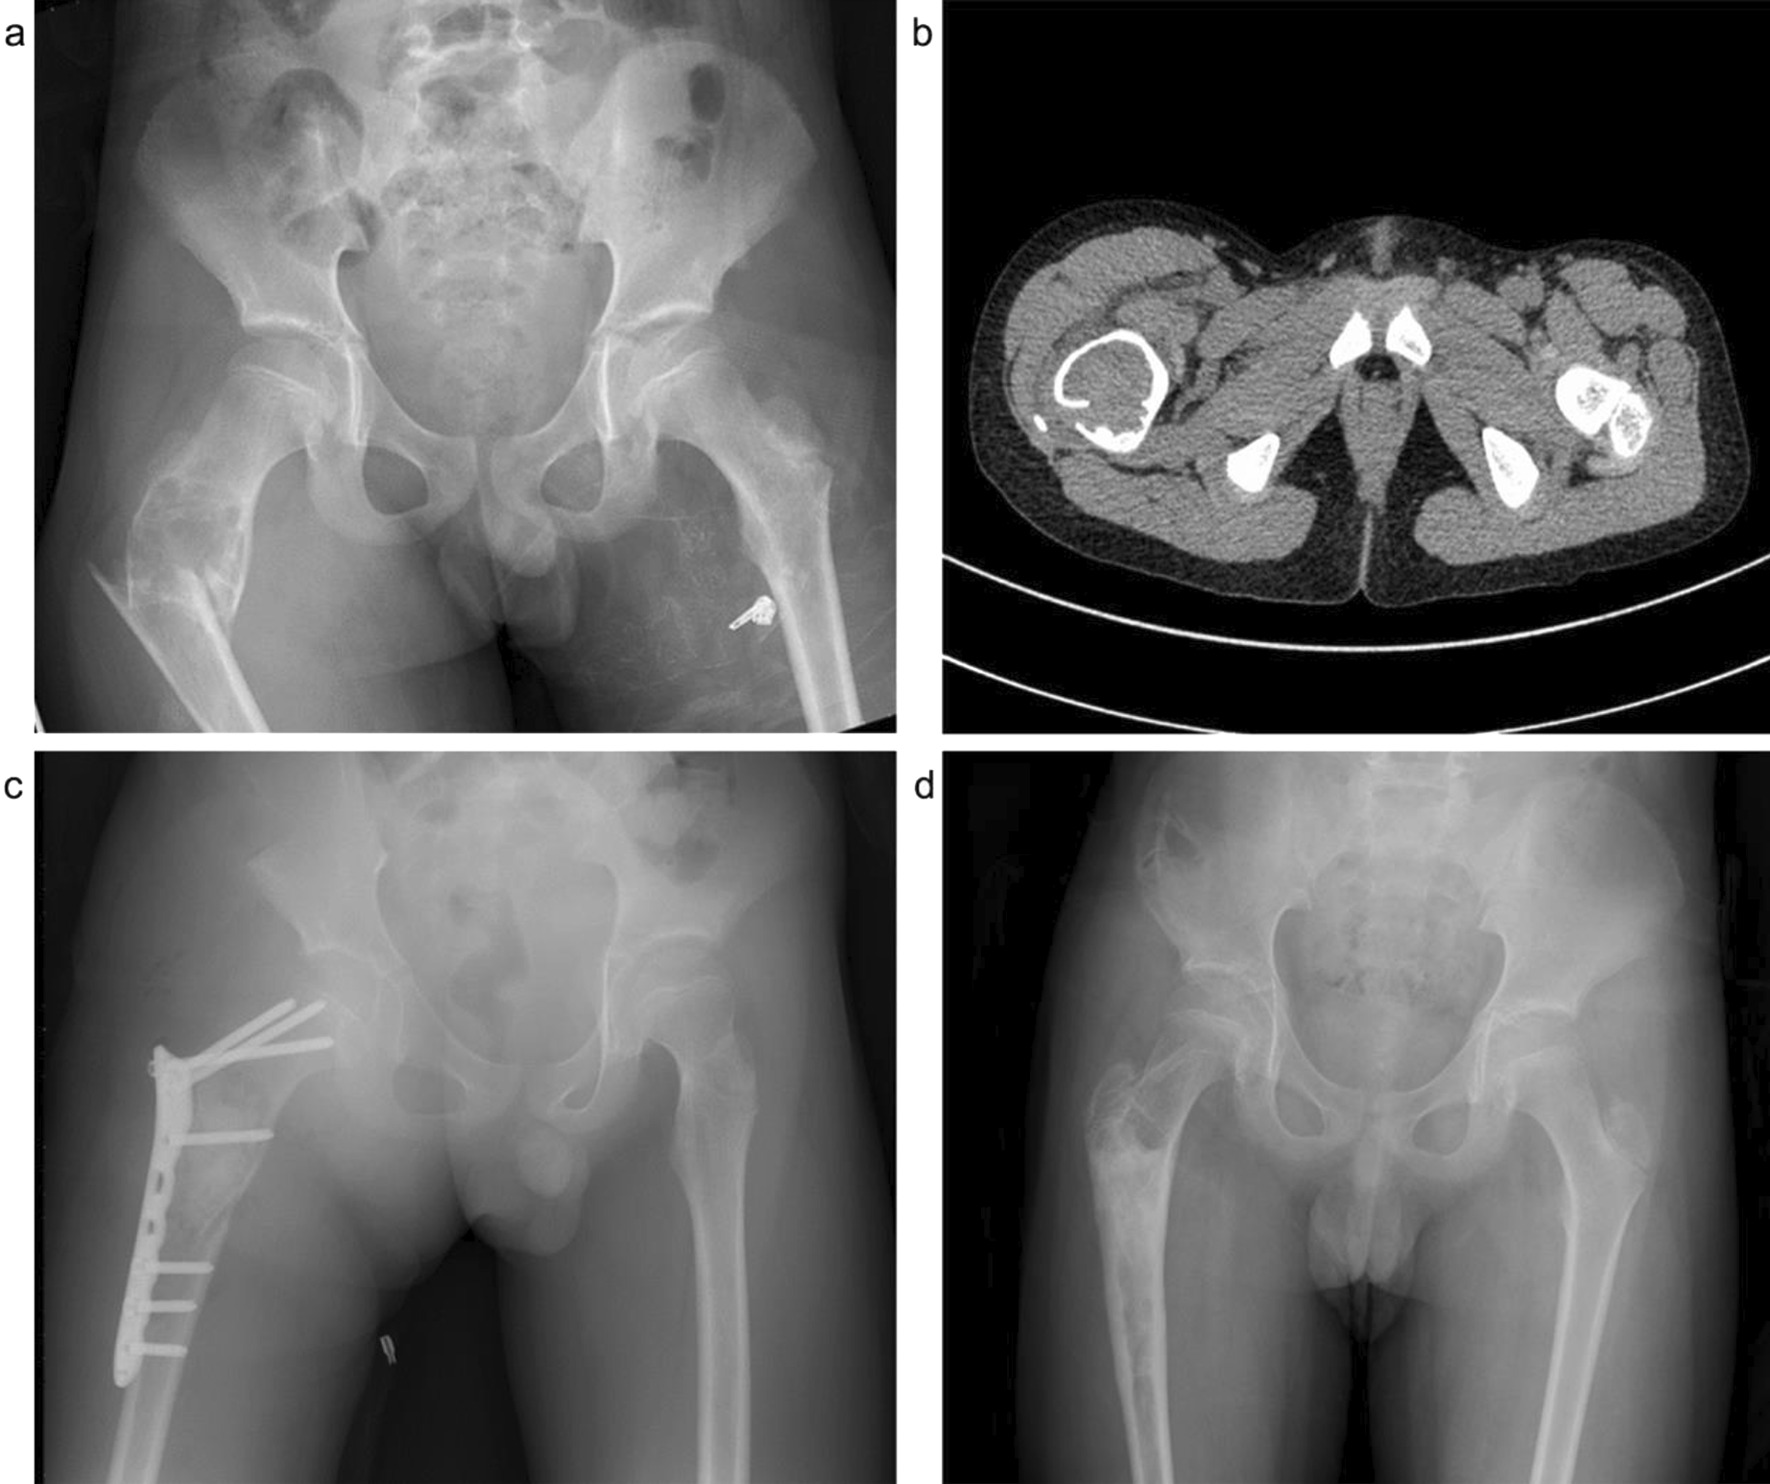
A 12-year-old boy diagnosed with simple bone cyst of right femoral trochanter with a pathological fracture. Surgical treatment of the right femur tumor was performed by curettage, artificial bone + autogenous iliac bone grafting; the pathological fracture was treated by orthopedic reduction and internal fixation with Locking Compression Pediatric Hip Plate™. a. Preoperative X-ray; b. Preoperative CT; c. Postoperative; and d. Two years after operation
